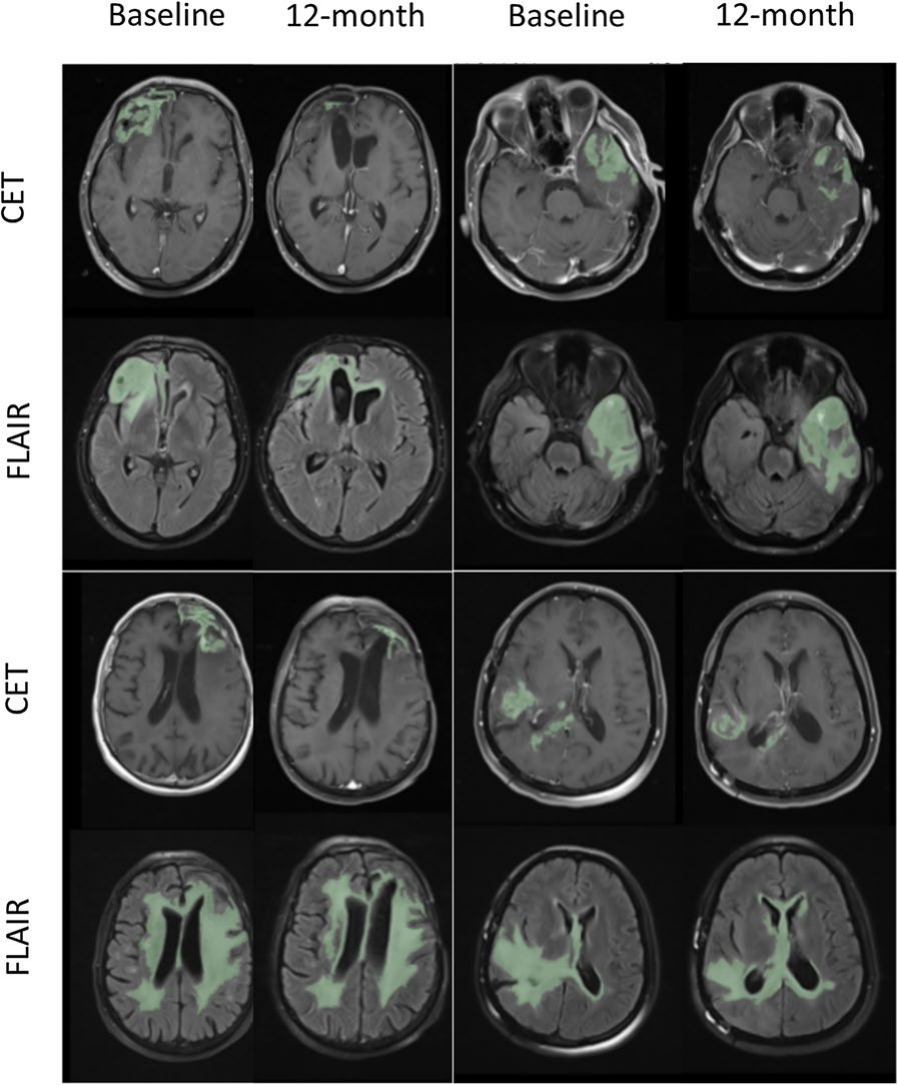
MR images, including post-gadolinium T1 weighted images and fluid attenuated inversion recovery (FLAIR) at baseline and 12-month after the suicide gene therapy using ADSC vehicles in four patients showing changes in contrast-enhanced tumor (CET) and FLAIR volumes
